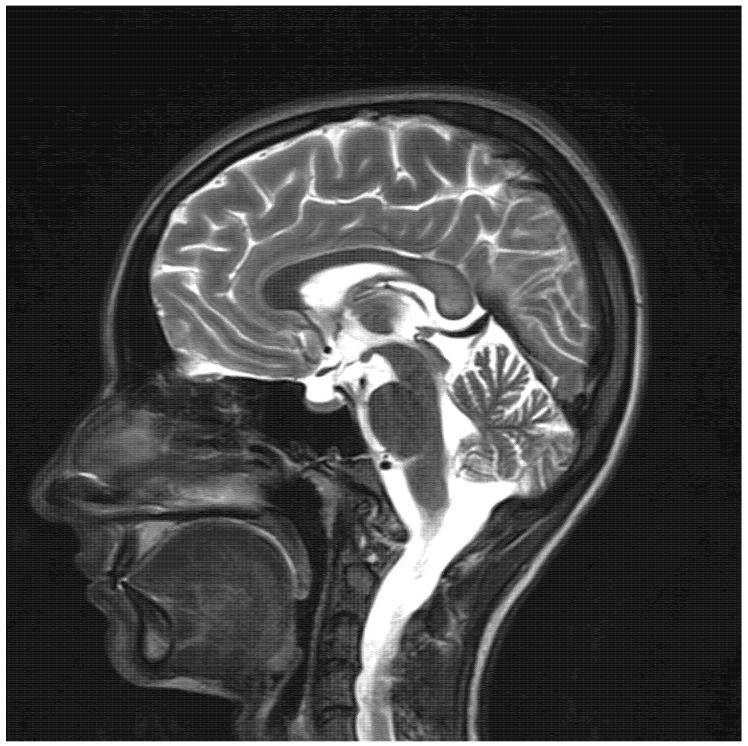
T2-weighted MRI sagittal view showing signal hyperintensity within the splenium of the corpus callosum.
